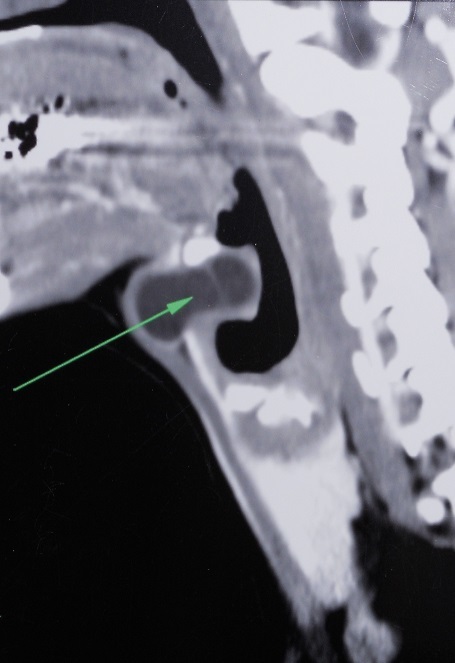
Cervical CT scann showing a bilobular cystic mass with intralryngeal extention
